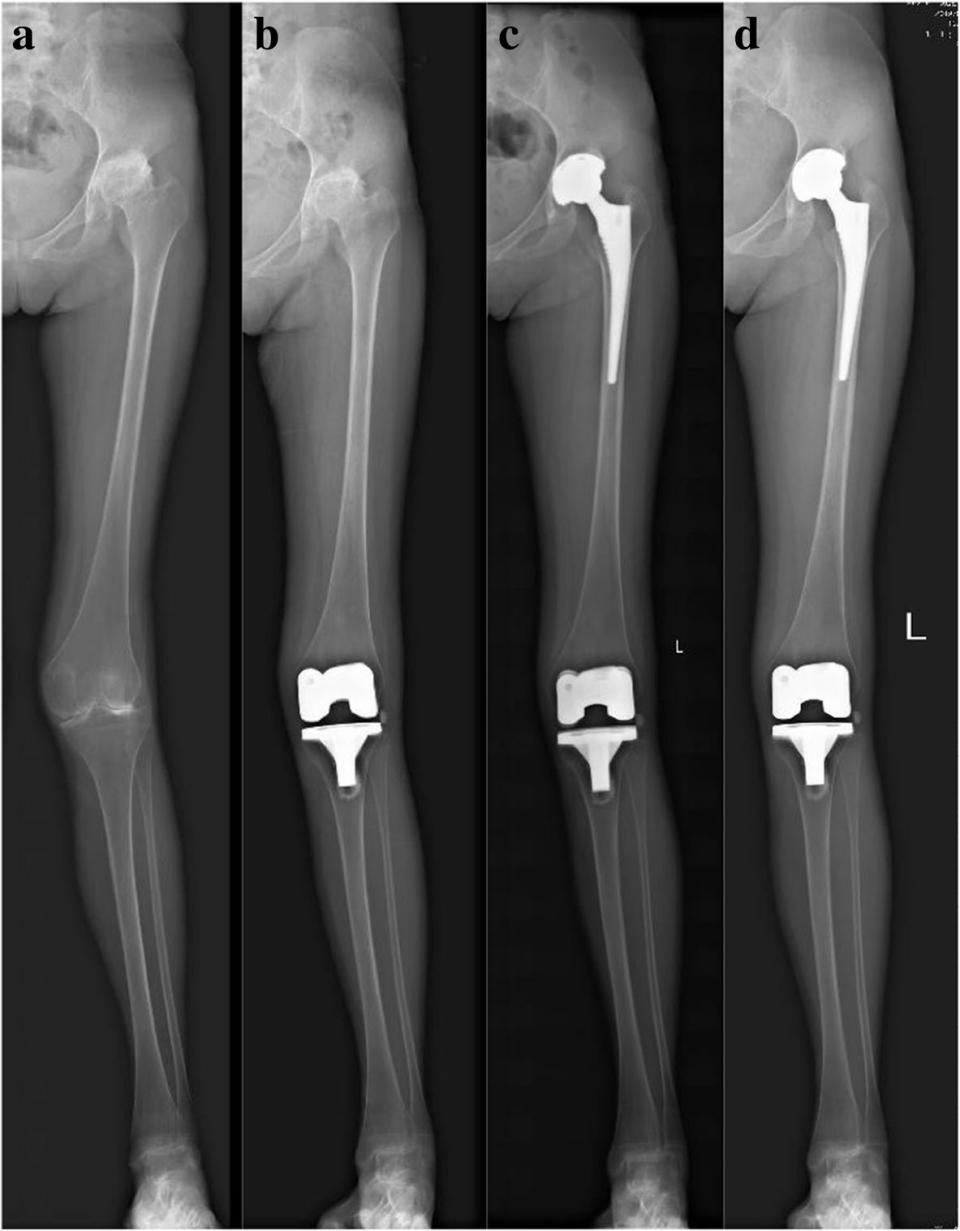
Radiographs (a-d) of a 27-y-old woman who underwent THA subsequent to ipsilateral TKA for rheumatoid arthritis. a Preoperative lower extremity image. b Radiograph of the patient undergoing TKA at 6-month follow-up. One year after prior TKA, the patient underwent ipsilateral THA. c The radiograph of the lower extremity at 6-month follow-up after THA showed stable implant fixation. d Postoperative radiograph of acetabular, femoral, and tibial components at 8-year follow-up showed stable implant fixation
